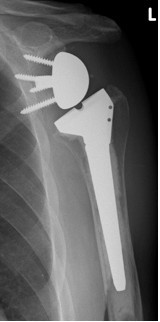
Antero-posterior radiograph of a 74 year-old female patient ´ s left shoulder with an implanted inverted total shoulder prosthesis Delta at 64 months of follow-up. Radiological analysis reveals with "grade 3 = erosion up to the inferior screw" of infraglenoidal scapular notching according to Nerot.
